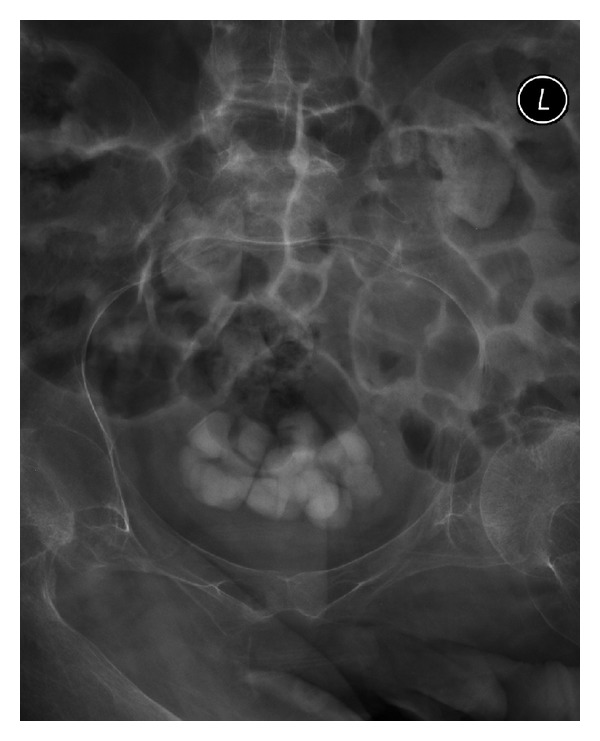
X-ray of abdomen, performed on 05 December 2008, revealed multiple calculi in urinary bladder.
